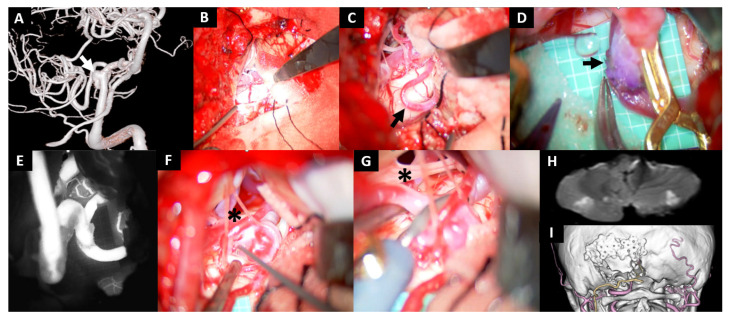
Case 10: A left VA–PICA aneurysm treated with OA–PICA bypass and trapping via the transcondylar fossa approach. (A) 3D-rotational digital subtraction left vertebral angiogram shows a small PICA aneurysm with a wide neck (arrow). (B–G) Intraoperative photographs. (B) Retraction of the cerebellum alone does not expose the distal PICA. (C) Opening the cerebellomedullary fissure allows wide exposure of the caudal loop of the PICA (arrow). (D) Intraluminal white thrombus is formed during the anastomosis (arrow) but resolved with gentle massage. (E) Patency of the bypass is confirmed by indocyanine green angiography. (F,G) The aneurysm and the origin of PICA is adjacent to the CN XII (*). Gentle retraction of the nerve by the assistant enables trapping with aneurysmal clips. (H) Postoperative MRI diffusion-weighted imaging shows ischemic changes in bilateral PICA region. (I) Postoperative 3D-reconstructed CT angiogram shows disappearance of the aneurysm and good patency of the OA-PICA bypass (yellow).Extracranial arteries unrelated to the bypass are indicated in pink.
